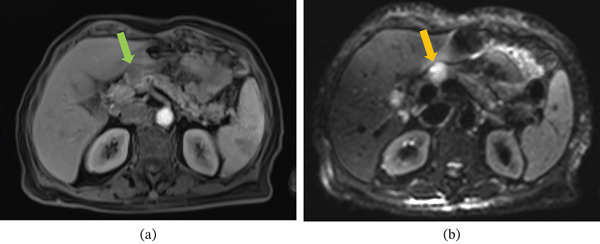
(a) Axial T1‐weighted MRI image demonstrating a 1.9‐cm hypoenhancing lesion in the pancreatic head (green arrow), consistent with a metastatic focus from uterine leiomyosarcoma. (b) Axial diffusion‐weighted MRI (DWI) image demonstrating restricted diffusion in the pancreatic head lesion (yellow arrow), consistent with a metastatic focus from uterine leiomyosarcoma.
